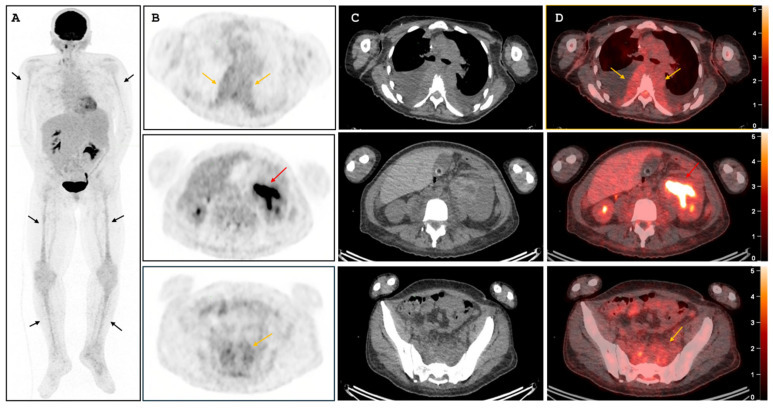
[^18^F]-FDG-PET/CT. (A) Maximum intensity projection (MIP) showing diffuse heterogeneous skeletal uptake, predominantly involving the long bones (black arrows), in keeping with peripheral redistribution of active haematopoiesis. (B) Axial PET images demonstrating low-grade uptake in the thoracic paravertebral masses (orange arrows, upper panel) and presacral lesion (orange arrow, lower panel). Evaluation of the left renal peripelvic/pelvicalyceal lesion (red arrow, middle panel) is limited by adjacent physiological urinary tracer activity. (C) Corresponding axial low-dose CT images. (D) Corresponding fused PET/CT images. To further characterize the CT abnormalities, [^18^F]-FDG-PET/CT was performed. It showed diffuse heterogeneous skeletal uptake, predominantly involving the long bones, suggesting redistribution of active haematopoiesis to the peripheral skeleton. The thoracic paravertebral and presacral soft-tissue lesions demonstrated only low-grade uptake, whereas the left peripelvic/pelvicalyceal lesion remained metabolically indeterminate because evaluation was limited by adjacent physiological urinary activity. This renal lesion raised an important diagnostic dilemma between upper tract urothelial malignancy and extramedullary haematopoiesis related to the underlying haematological disorder.
